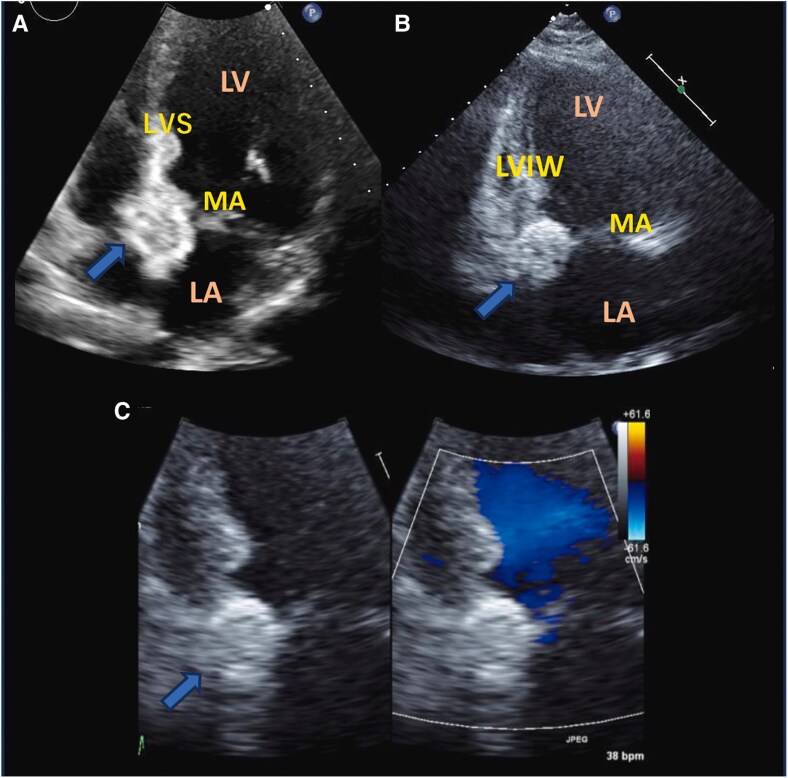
Transthoracic echocardiogram at presentation: the apical four-chamber view (*A*) and the apical two-chamber view (*B*). The mass has a high-intensity area of margins and an echolucent area of intensity, and there is no colour signal (*C*). LA, left atrium; LV, left ventricle; MA, mitral annulus; LVS, left ventricular septum; LVIW, left ventricle inferior wall.
